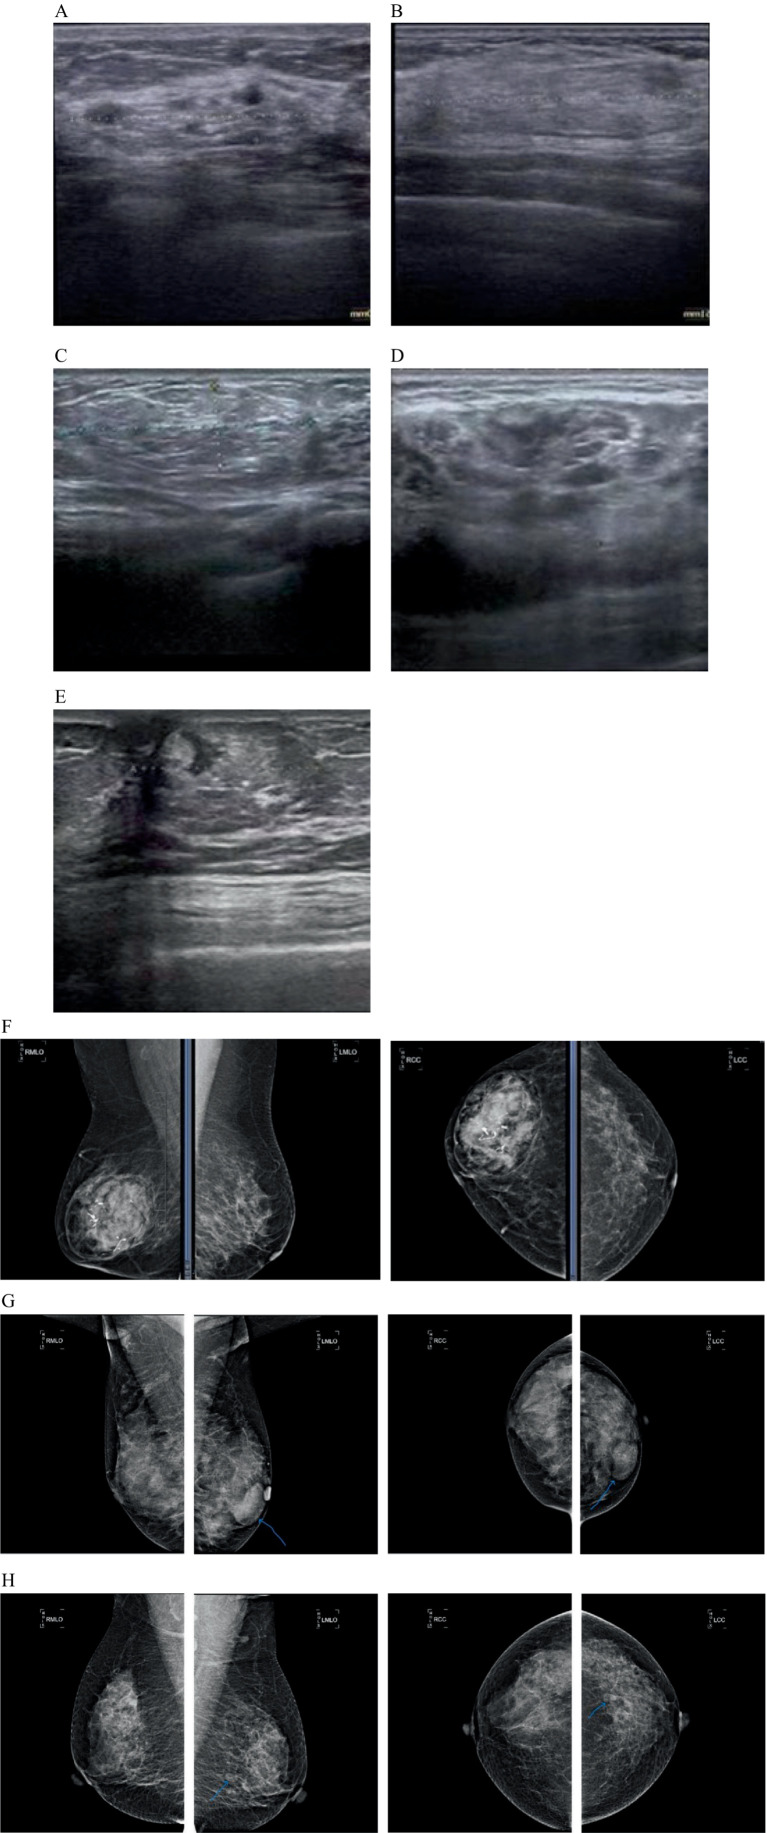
The characteristics of breast hamartoma on color Doppler ultrasound and mammography (breast X-ray imaging). (A) Ultrasound image showing a mixed echo mass. (B) Ultrasound image showing an iso-echo mass. (C) Ultrasound image showing an uneven echo mass of fat. (D) Ultrasound image showing a hypoechoic echo mass. (E) Ultrasound image showing a slightly strong echo mass. (F) Mammogram showing an oval mass of high, equal and low mixed density with clear margin and coarse calcification in the right breast. (G) Mammogram showing multiple iso-density mass in the left breast, the larger one is in the inner lower quadrant, about 4.0×2.6 cm in size, the boundary is clear. (H) Mammogram showing round-like low-density shadows in the lower outer quadrant of the left breast.
